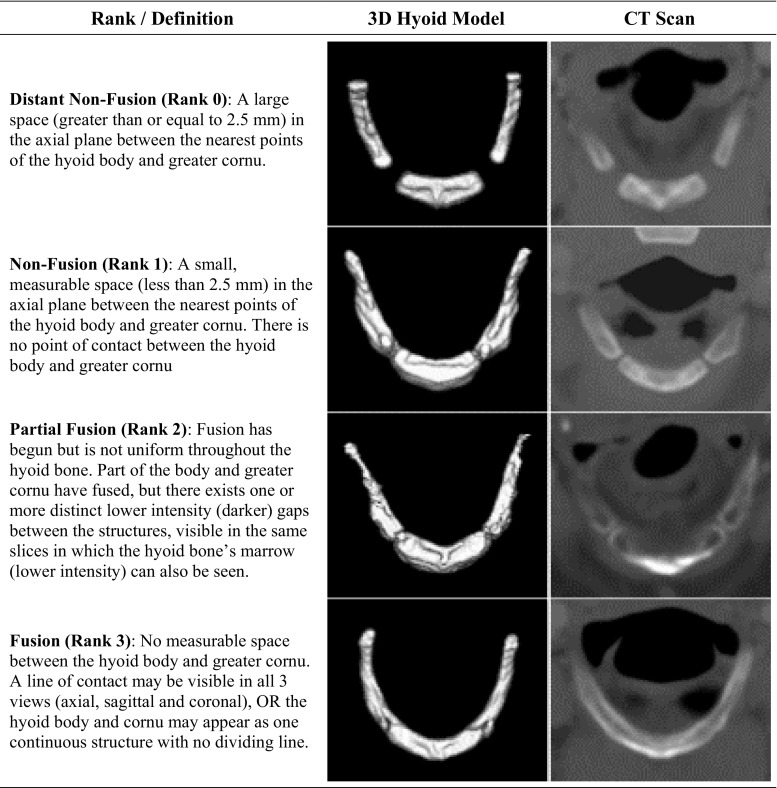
Fusion rank definition with images showing the same fusion rank bilaterally. The 3D Hyoid bone models were created using image visualization and analysis software Analyze 11.0^®^ (AnalyzeDirect^®^; Overland Park, KS). The axial slice from the CT scan with the smallest distance between the hyoid body and the greater cornua was used for fusion ranking but all axial, coronal, and sagittal views were inspected to accurately determine degree of fusion
